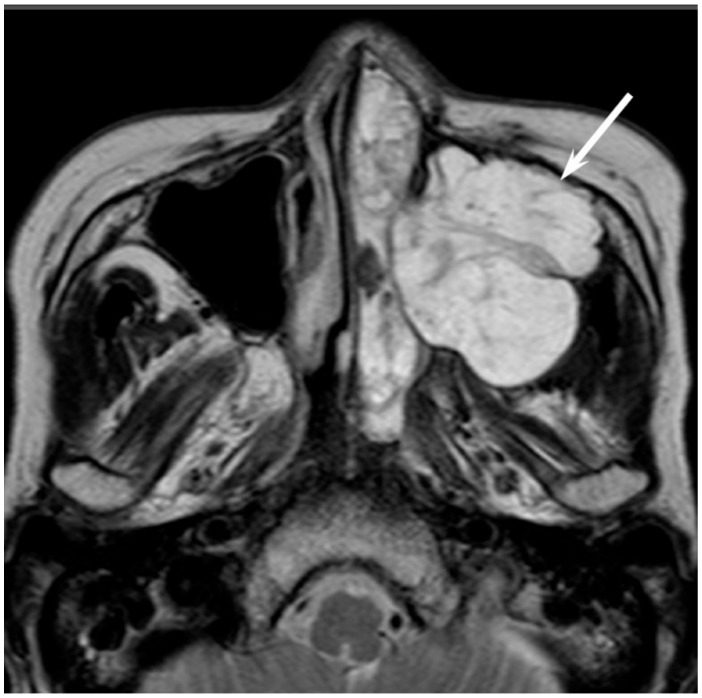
Adenoid cystic carcinoma of the left maxillary sinus and nasal cavity. T2-weighted image showing a well-demarcated, lobulated, heterogeneously and strongly hyperintense lesion (arrow).
